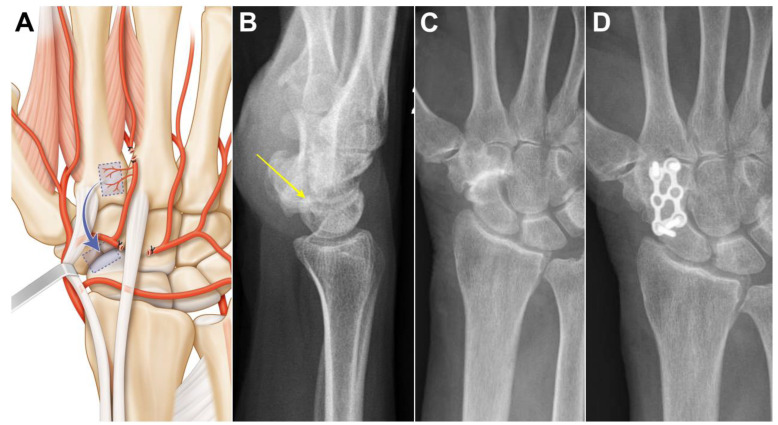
Case 1. (A) Arterial anatomy, the radially based pedicle necessitates ligating distal and ulnar branches; (B,C) preoperative X-ray showing advanced scaphotrapeziotrapezoidal arthritis and no DISI (yellow arrow); (D) union is obtained in less than three months.
